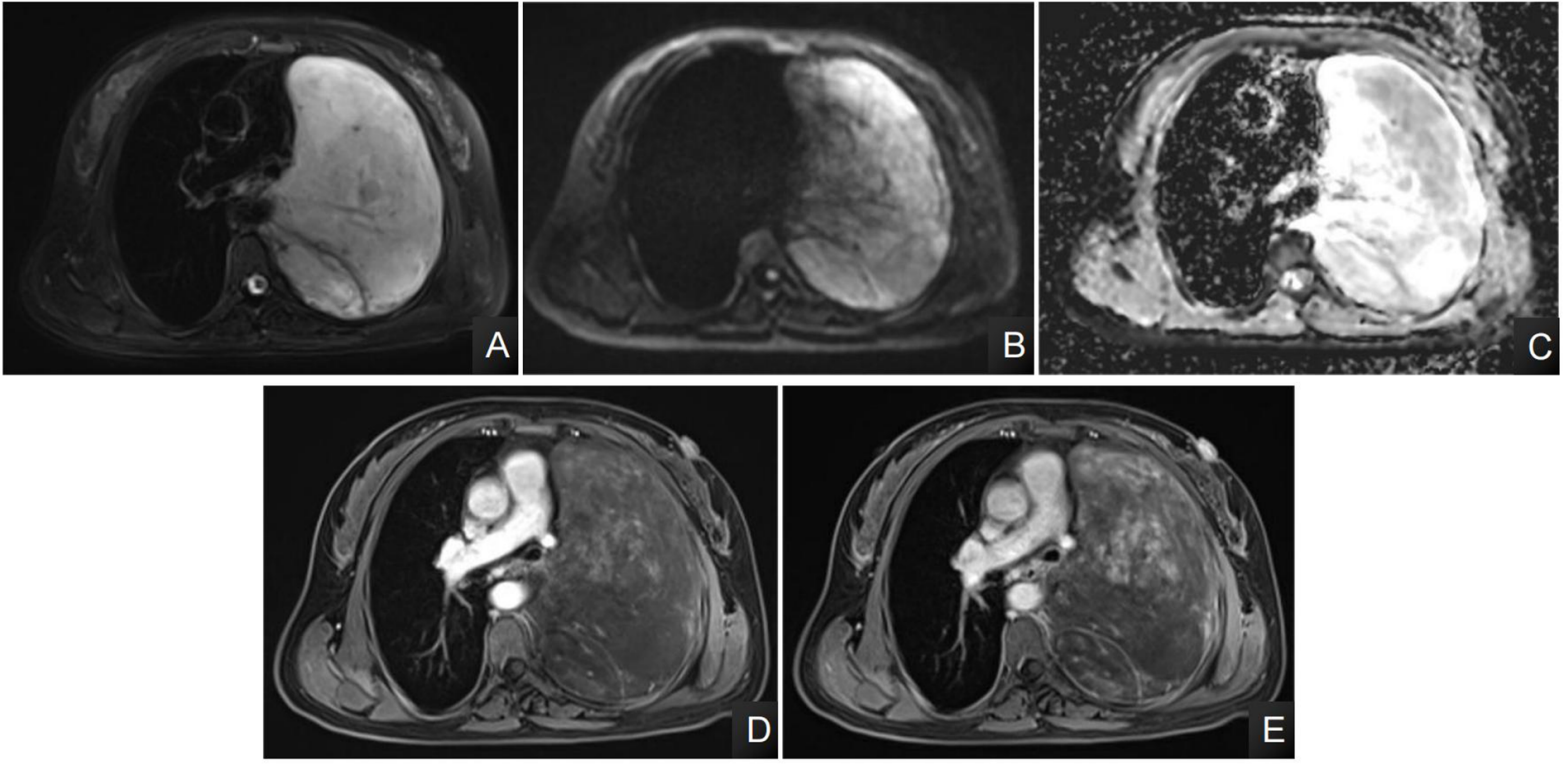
(A–E) The axial T2-weighted MR images reveal an uneven high signal with a “streaky” appearance (A); DWI images showed partially high signal, and ADC images showed a slightly low signal in the ADC in the area corresponding to the high signal in DWI (B, C). The axial MRI enhancement image showed mild to moderate heterogeneous delayed enhancement of the lesion (D, E).
